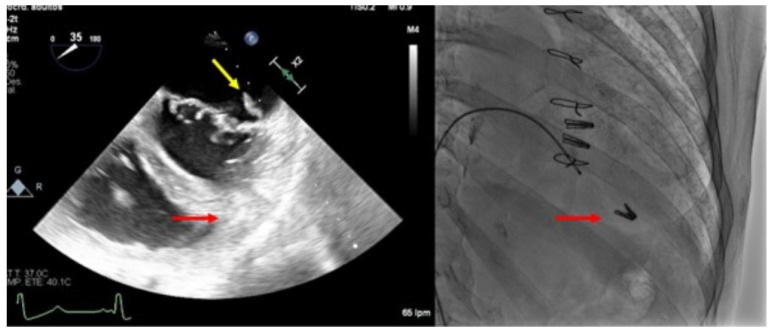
Clip embolization. (Left) Two-dimensional transesophageal echocardiogram showing a posterior leaflet prolapse (yellow arrow) after retrograde embolization of a clip to the right ventricle through the procedural atrial septal defect (red arrow). (Right) Fluoroscopy image at the end of the procedure showing two clips implanted in the mitral valve and another one embolized in the apex of the right ventricle (red arrow).
